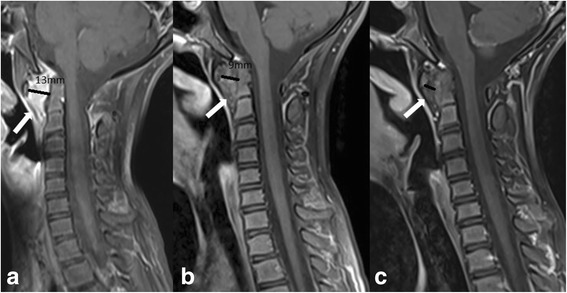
MRI of cervical spine (patient #8)(T1 TSE FS sagittal with a contrast medium): a initial MRI (January, 2013) showed an intense contrast enhancement of a huge synovial thickening (white arrow) in predental space with a huge anterior atlanto-axial subluxation (aAAS)(black line, 13 mm) and a compression of the anterior liquor space, normal shape of the dens, b follow-up MR (January, 2014): the evaluation of the treatment with anti-TNFα showed a reduction of synovial thickening and a less intense contrast-enhancement and less significant aAAS, normal width of the anterior liquor space, but with initial morphological changes of the dens, c follow-up MRI (March, 2016): the evaluation of the treatment with anti-TNFα showed a significant reduction of synovial thickening in the predental space and aAAS, only patchy contrast enhancement, but with morphological changes of the dens and a thinned corticalis
